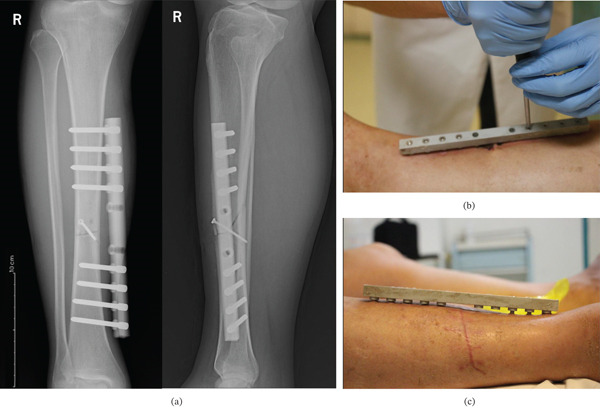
(a) Anteroposterior (left) and lateral (right) radiographs of the tibia at the 4‐week follow‐up showing early callus formation. (b, c) Removal of the two screws closest to the fracture site 1 month postoperatively.
